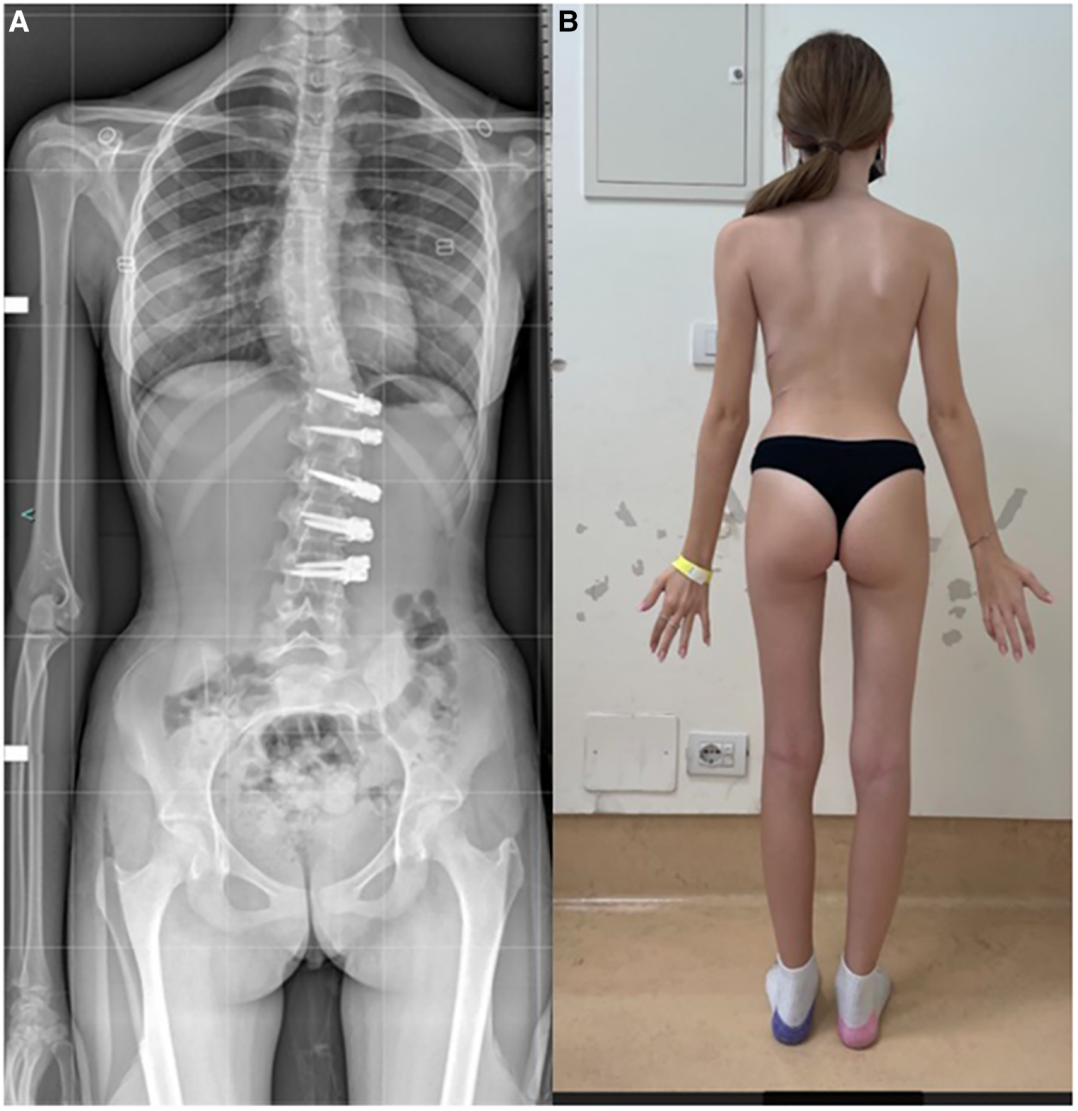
6-months post-tethering; (A) radiographically, Cobb angle maintained at 17°, (B) patient is apparently well balanced.
